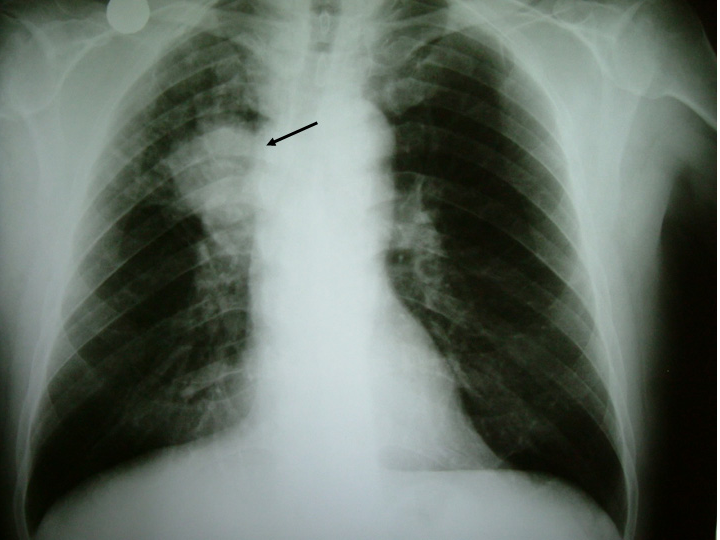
The x-ray finding of the bronchogenic carcinoma.
